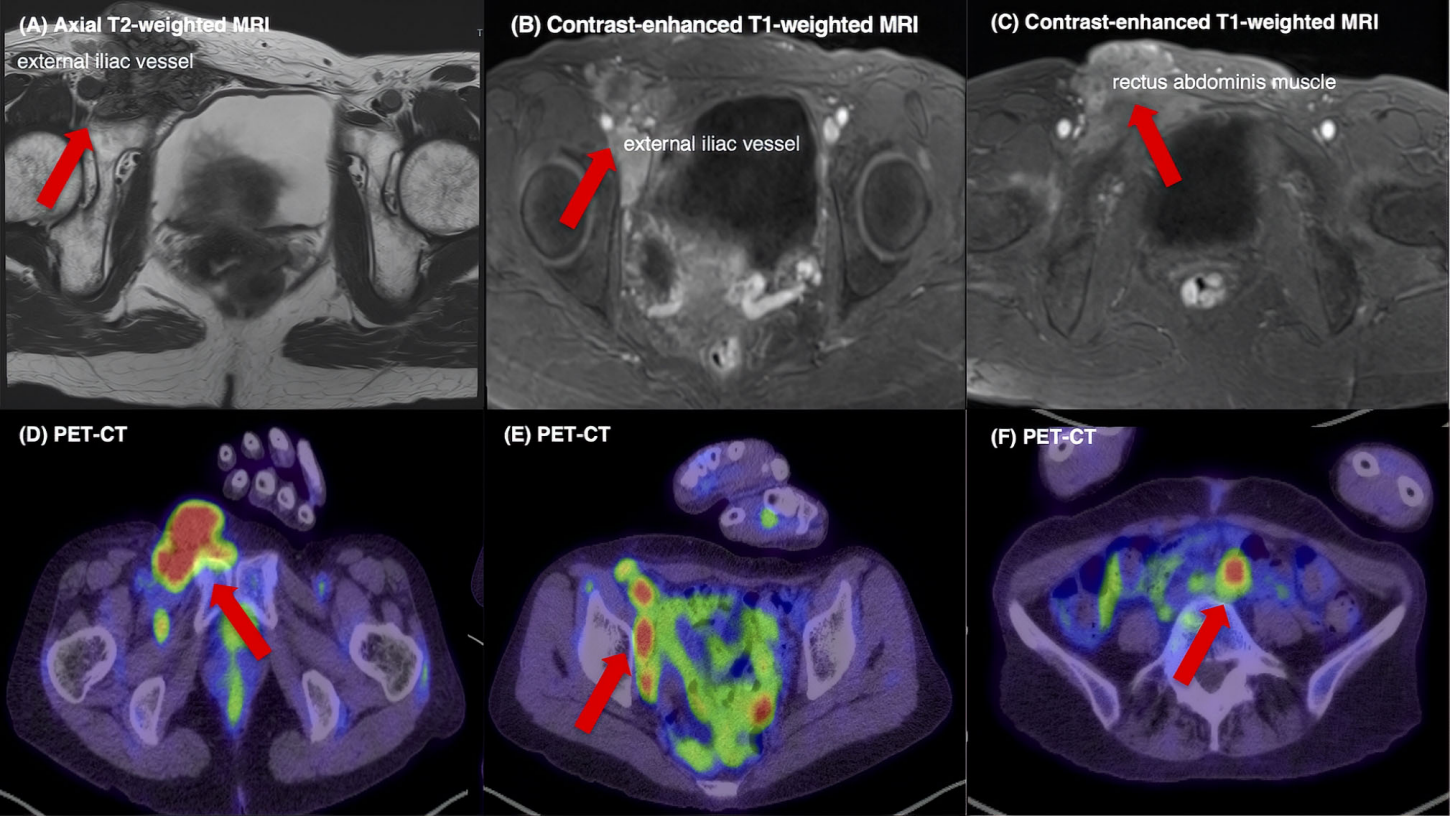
Preoperative MRI and PET-CT findings. (A) Axial T2-weighted MRI showing a heterogeneous 9 cm mass in the right inguinal–pelvic region with extension toward the external iliac vessels and infiltration of the lower abdominal wall. (B) Contrast-enhanced T1-weighted MRI demonstrating focal involvement and displacement of the external iliac/femoral vein. (C) Contrast-enhanced T1-weighted MRI showing linear enhancement along the rectus abdominis muscle, consistent with anterior abdominal wall infiltration. (D) PET-CT maximum-intensity projection and axial fusion images showing high FDG uptake in the right inguinal lesion, together with (E) multiple FDG-avid pelvic lymph nodes and (F) para-aortic lymph nodes, indicating extra-pelvic disease extension consistent with systemic nodal metastases. FDG uptake in the bilateral ovarian tumors was also observed but is not shown.
